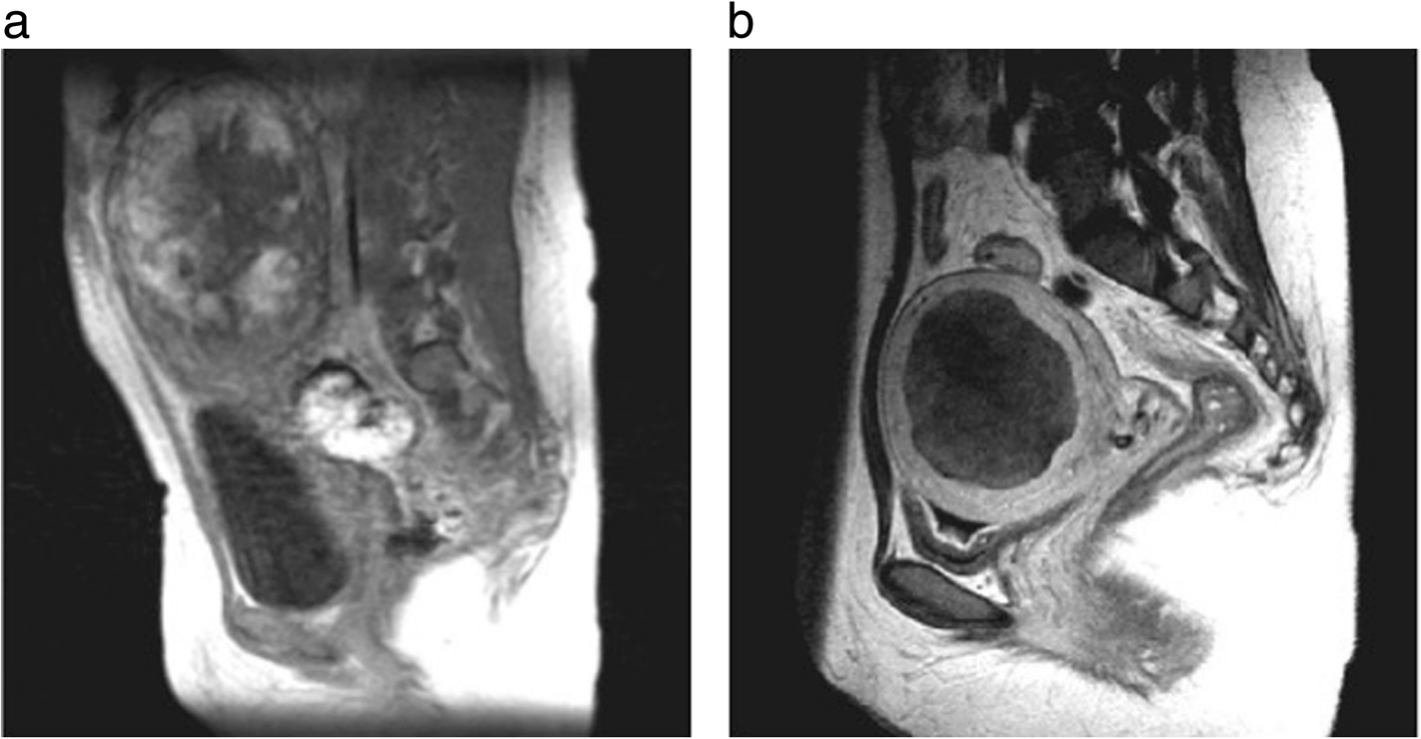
a Magnetic resonance imaging scan taken 7 days post-partum. Myometrial invasion by placental tissue is shown and the whole placenta (13.4×11.6×8.6cm) was strongly enhanced. b After 13 courses of methotrexate therapy, placental tissue was reduced and none of the placenta (7.0×6.8×6.6cm) was enhanced
